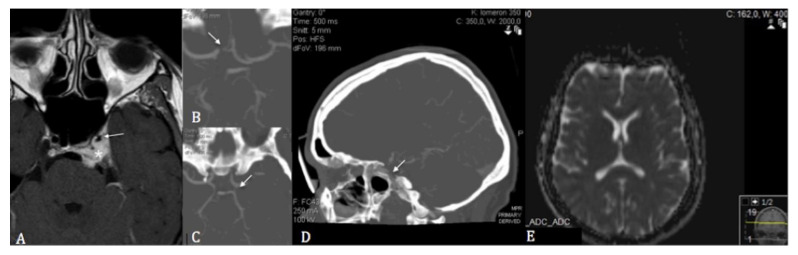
(A) T1 gadolinium-enhanced axial cerebral magnetic resonance imaging showing the encasement of the left internal carotid artery (asterisk) with subsequent stenosis of its cavernous segment (white arrow) following stereotactic radiosurgery. (B) Axial computed tomographic angiography showing the hypoplastic pre-and post-communicating segments of the right anterior cerebral artery. (C) Axial computed tomographic angiography showing the left hypoplastic posterior communicating artery. (D) Sagittal computed tomographic angiography showing the stenosis of the cavernous segment of the left internal carotid artery. (E) Diffusion-weighted sequences showing no restriction of diffusion on admission magnetic resonance imaging.
